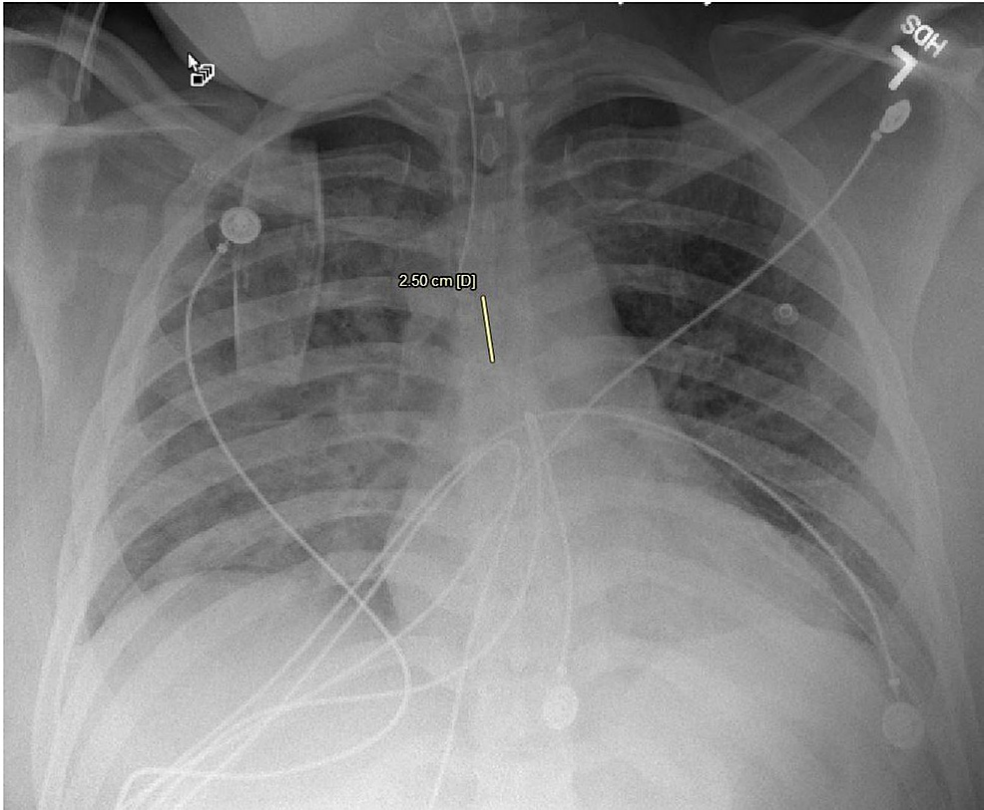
Post-intubation chest X-ray.
